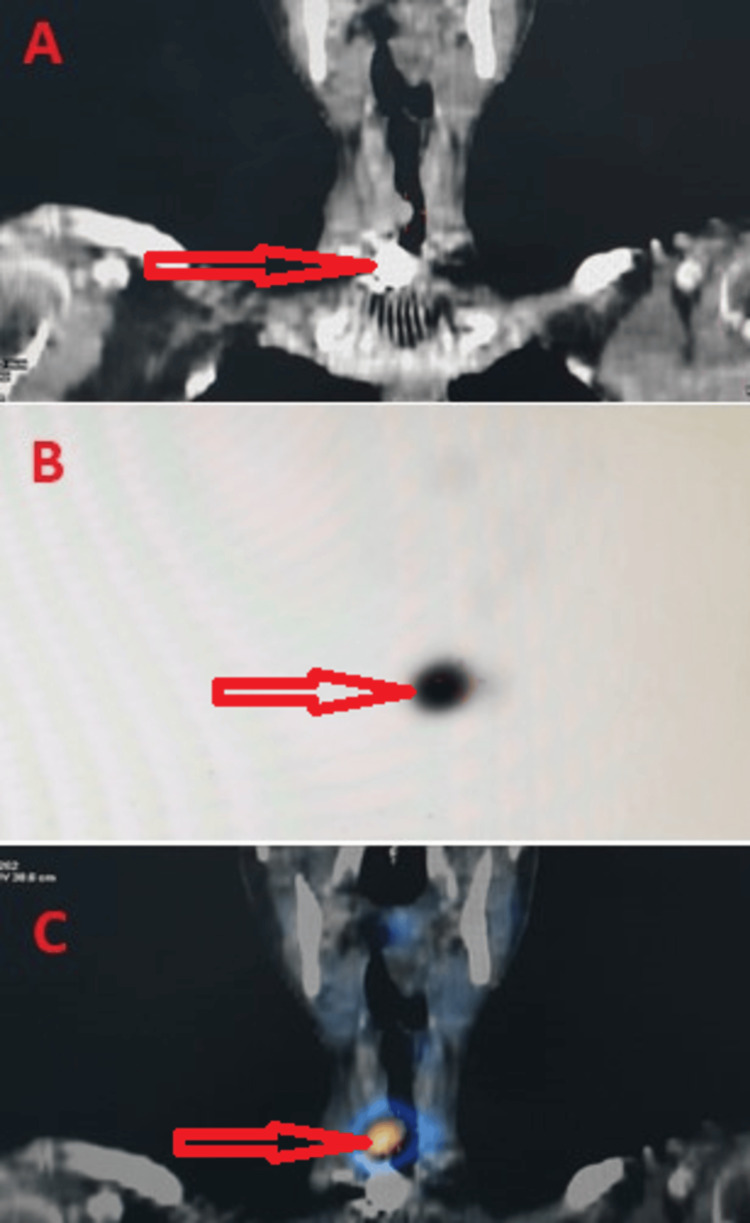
Coronal CT cervical image (A), SPECT 131I image (B), and fusion SPECT/CT image (C) showing intense uptake at the intra-tracheal pathological process. SPECT: single photon emission computed tomography.
